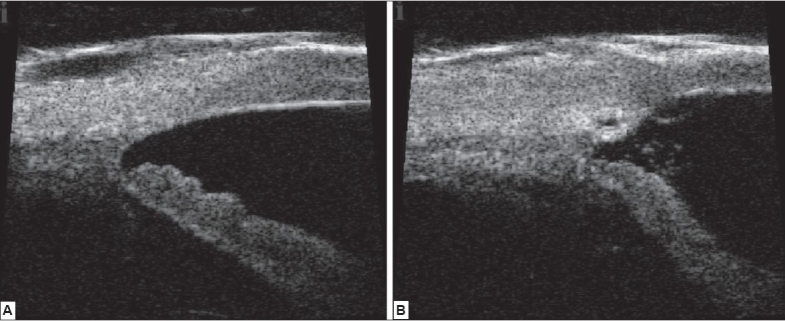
Images Captured with iUltrasound System: Same Eye before (A) and after (B) Canaloplasty. Increased Diameter of Schlemm's Canal postoperatively.
